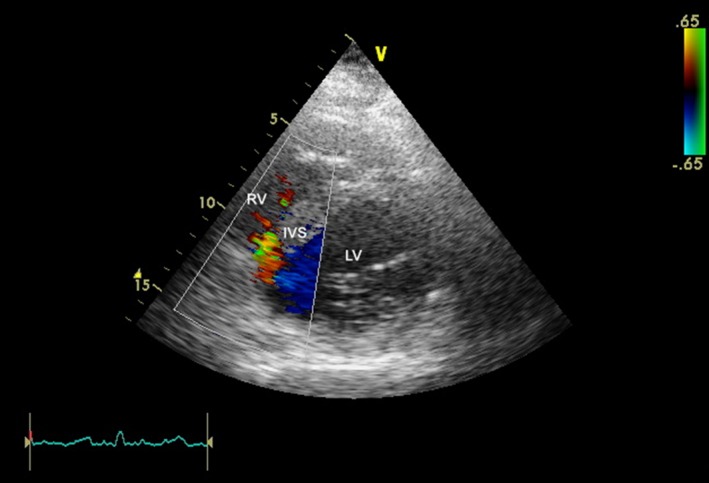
Presence of ventricular septal defect as seen in 2‐dimensional transthoracic echocardiography in parasternal short‐axis view (PTSAX)
